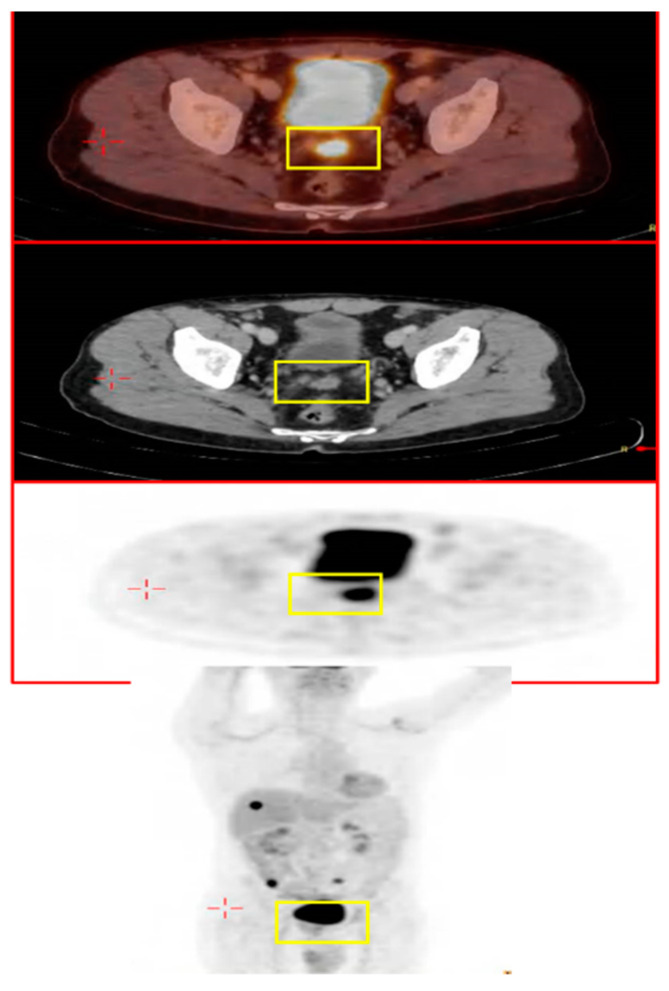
Images of the patient’s PET-CT showing the hyper-metabolic uptake in the area of the prostate (yellow squares).
